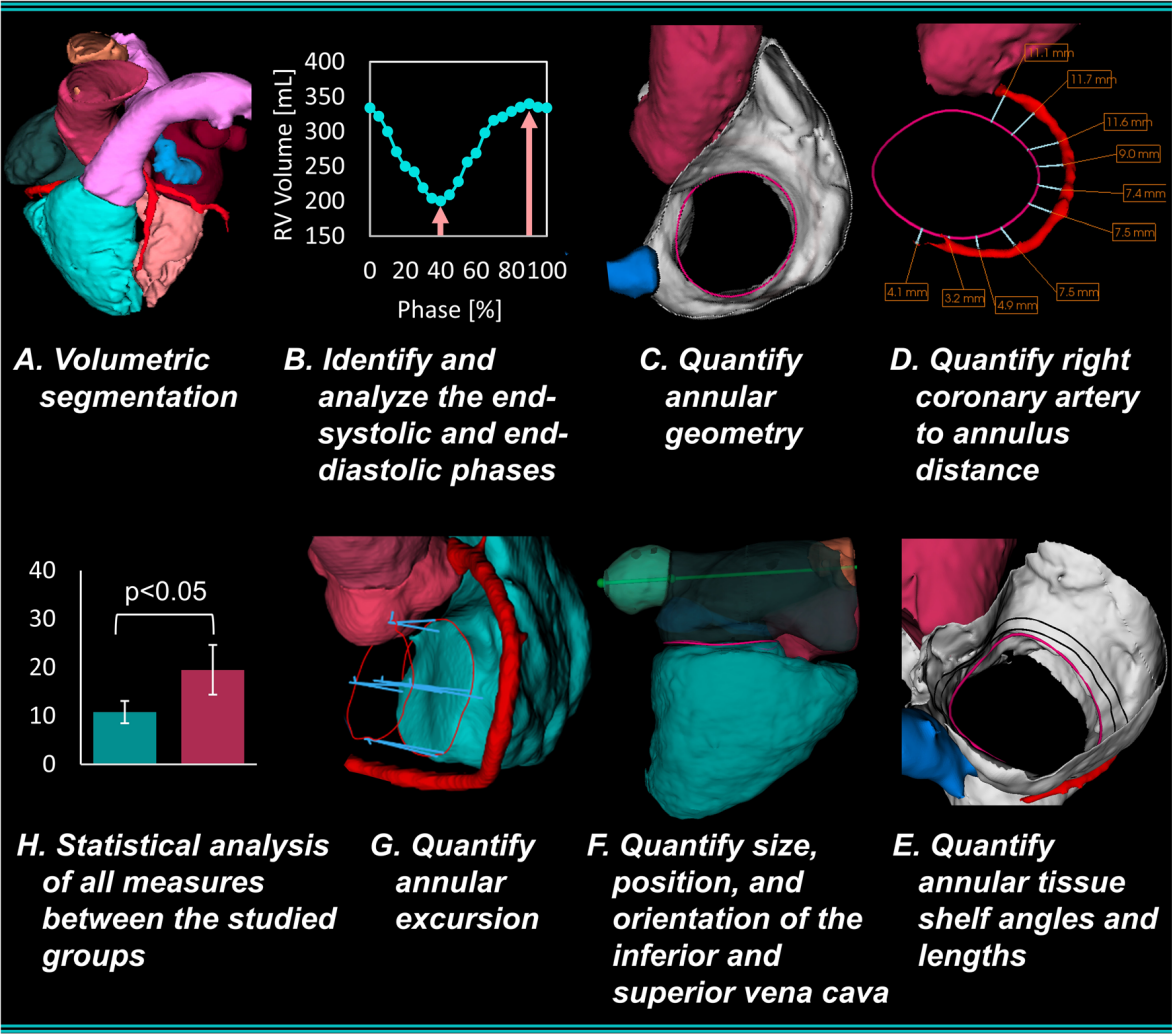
Cardiac computed tomography angiography analysis overview.
